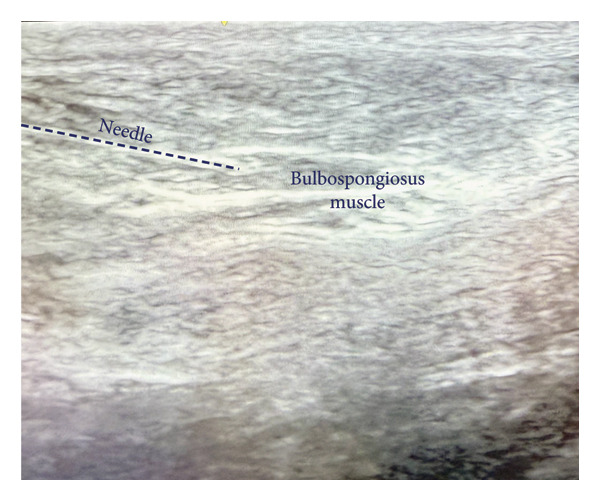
Ultrasound‐guided TPI of the bulbospongiosus muscle. *Source:* Alopi Patel, MD, 2025.
